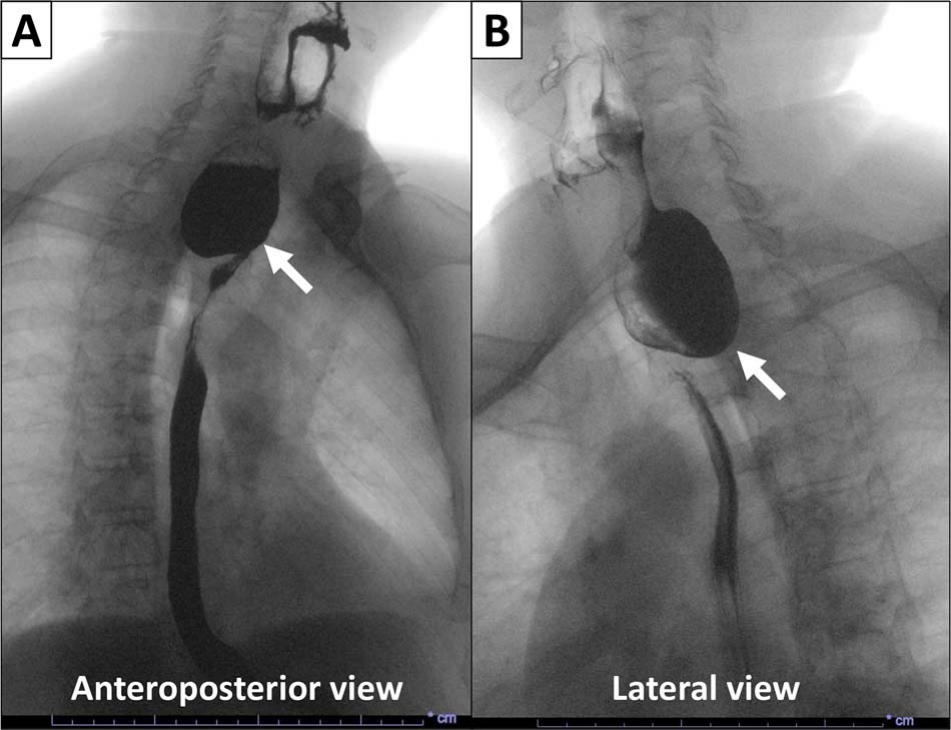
X-ray esophagogram performed in 2015. Esophagogram with barium suspension showed a 4 × 5 cm Zenker’s diverticulum with a 1.7 cm wide diverticular neck.
